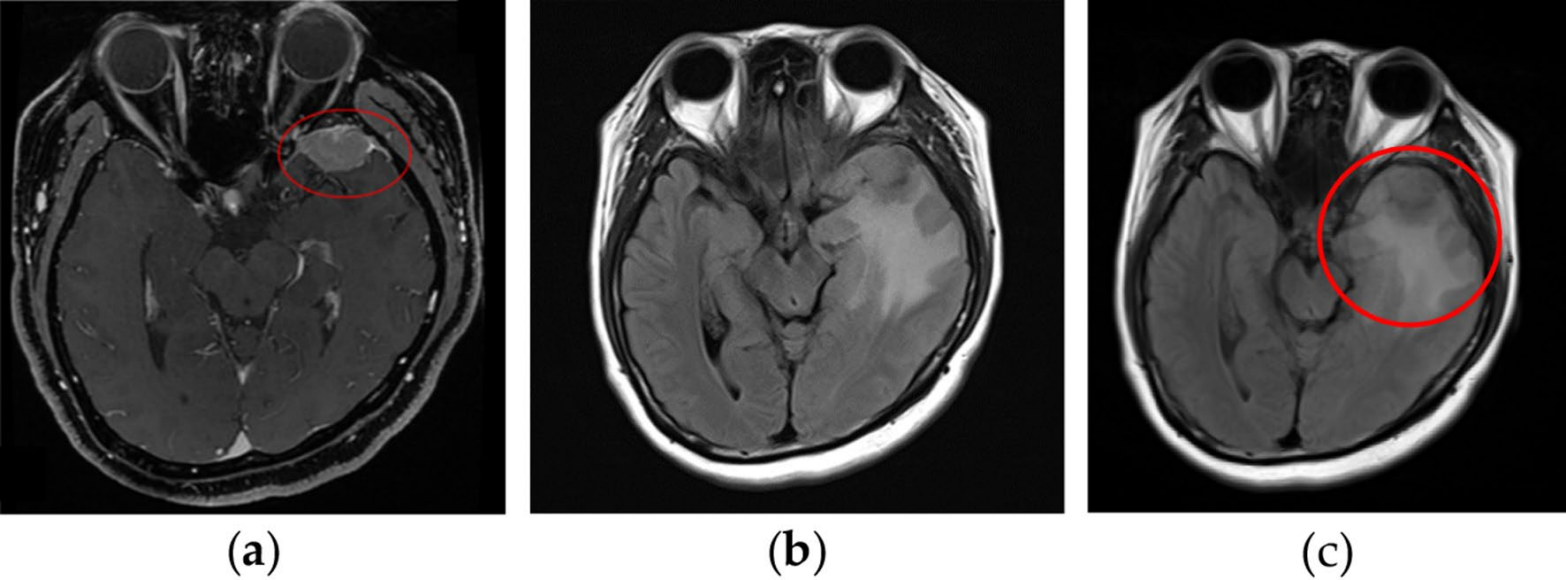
MRI images before treatment and MRI images after image fusion. (a) MRI before treatment. (b) MRI 4 months after treatment. (c) Cerebral edema area with fusion technology. *MRI* magnetic resonance imaging. Image source statement: This image was created using CyberKnife M6 system. Software URL: https://www.accuray.com/resources/accurays-cyberknife-m6-series/.
